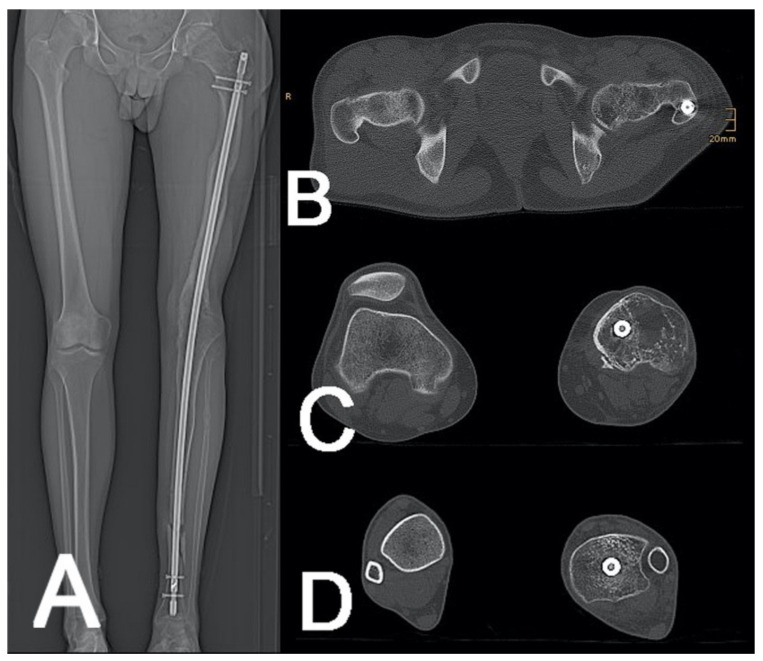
Rotational CT to plan the de-rotation surgery of a knee arthrodesis nail (A). Measurements of the hip (B), knee (C), and ankle joint (D) were performed.
